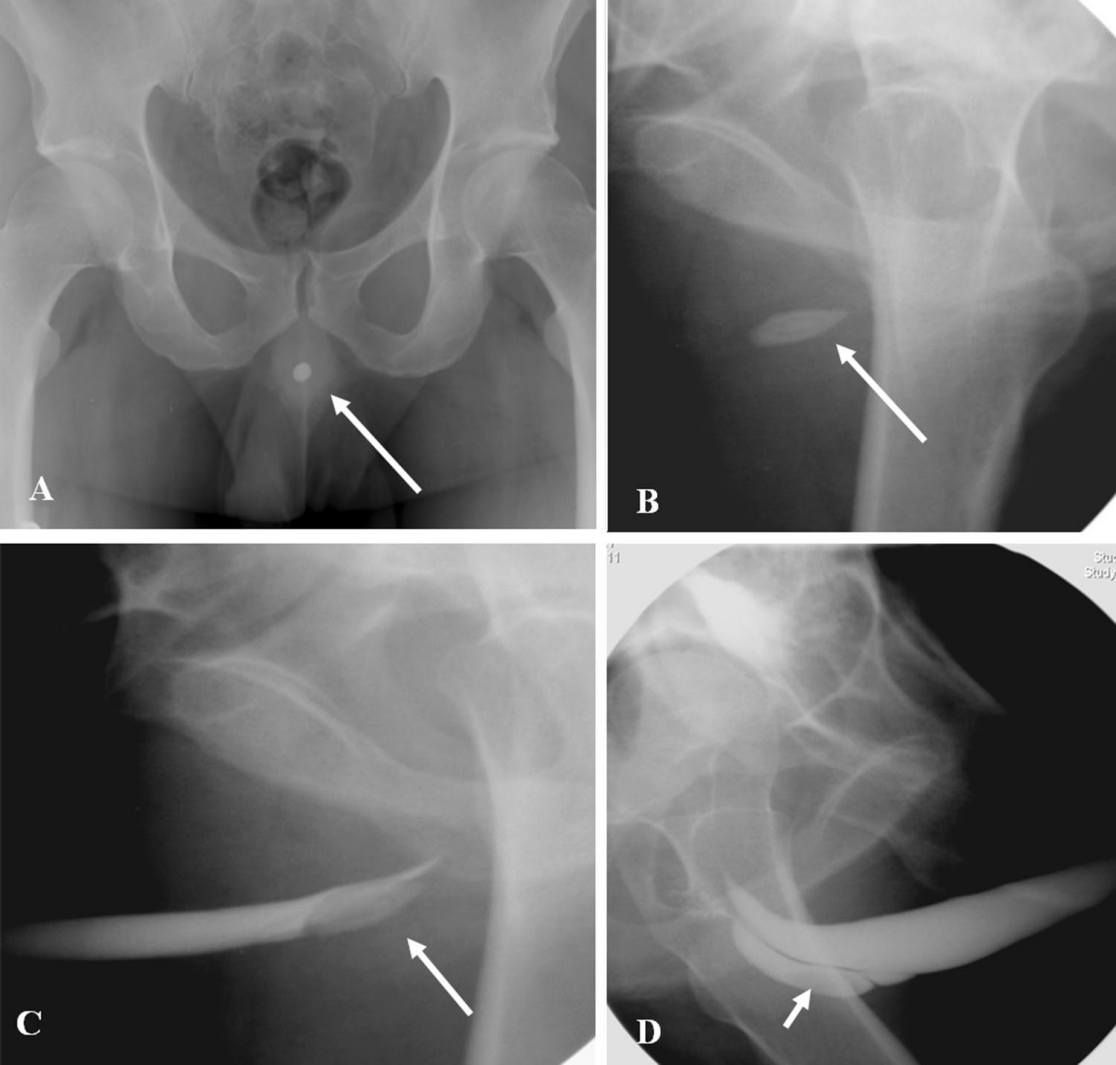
Calculus in Cowper’s duct syringocele. a, b Frontal and oblique plain radiograph shows radio opaque focus just below pubic symphysis along urethral course (arrows). c, d Retrograde urethrogram shows the calculus along the ventral aspect of proximal bulbar urethra (arrow) with extrinsic indentation. On further opacification (d), it is masked by the dense contrast in the dilated Cowper’s duct suggesting syringocele (short arrow)
